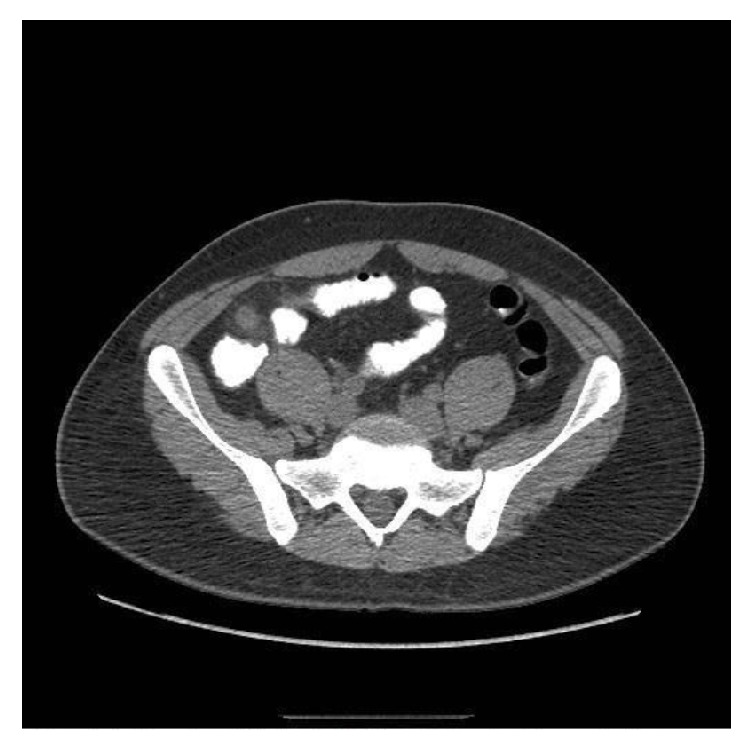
Axial section of abdominal CT scan with contrast demonstrating swollen appendix with 9.8 mm diameter, no appendicolith present.
